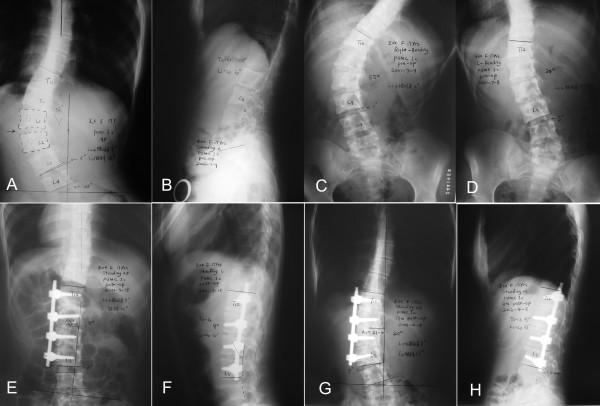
A 17-year-old female, PUMC Ic type. Preoperative X-ray showed a 52° left lumbar curve with the apex at L_1,2 _disc(A, B). The interspace angle between L_3 _and L_4 _was -5°. On the right Bending film, the interspace angle was 0°(C). On the left Bending film, it was still -5°(D), and T_12_-L_1_and L_2,3 _disc didn't open, so the fusion should include T_12 _to L_3_. Postoperative films showed a good correction and the interspace angle improved to 0° (E, F). Nine months later, there were some loss of the correction and the interspace angle increased to 7° (G, H).
